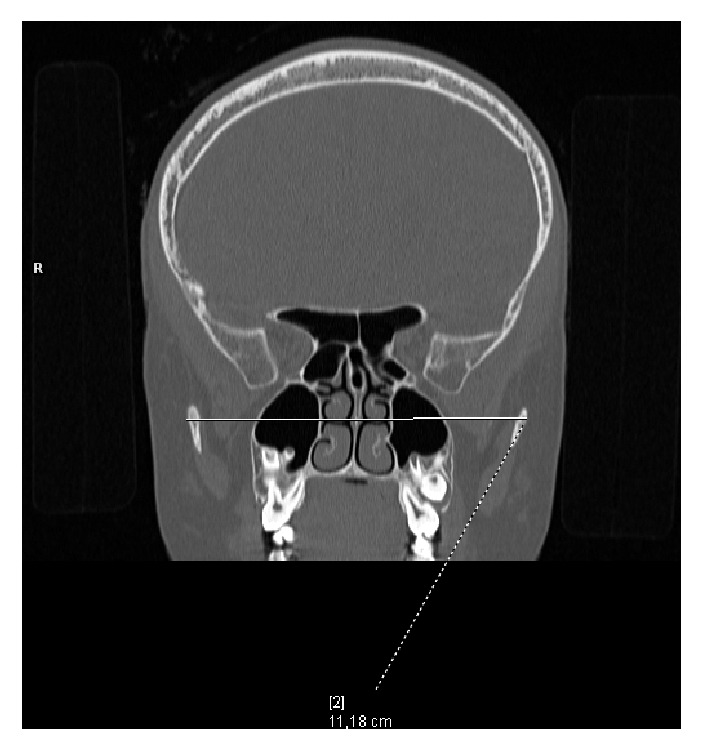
An example of zy/zy distance marked on the CT image.
